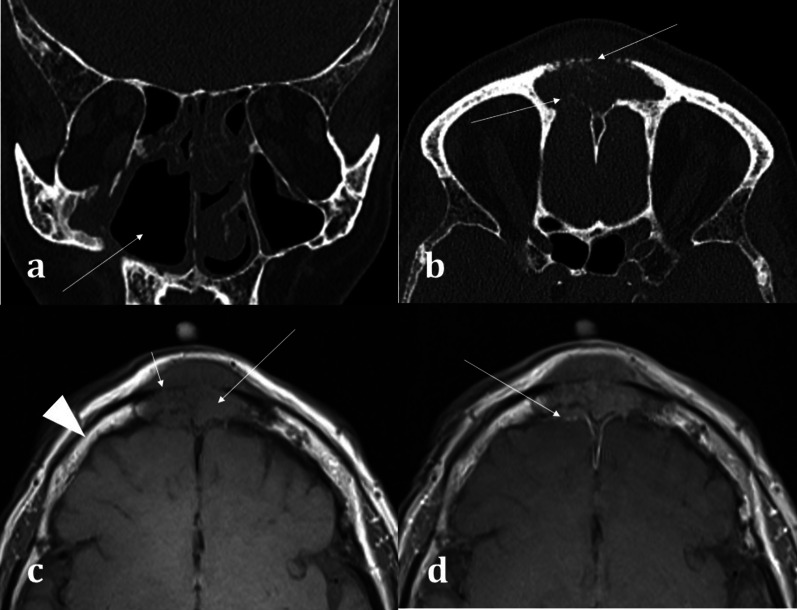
Transpatial spread of recurrent of sinonasal squamous cell carcinoma (SCC), in the same patient as shown in Fig. 4b. Coronal bone window CT a demonstrates the right maxillectomy. Axial bone window CT b shows a permeative process within the frontal sinus causing cortical destruction (arrowed). T1WI demonstrates recurrent SCC involving the frontal sinus (c), expanding the sinus and infiltrating the bone (long arrow) with loss of the normal fatty marrow signal (arrowhead for comparison). There is clear evidence of inner and outer table cortical breach on T1WI with loss of the normal hypointense cortical rim (short arrow) placing the patient at risk of intracranial disease. The lesion demonstrates mild enhancement (d), with associated dural thickening and enhancement (arrow) indicating transpatial spread
